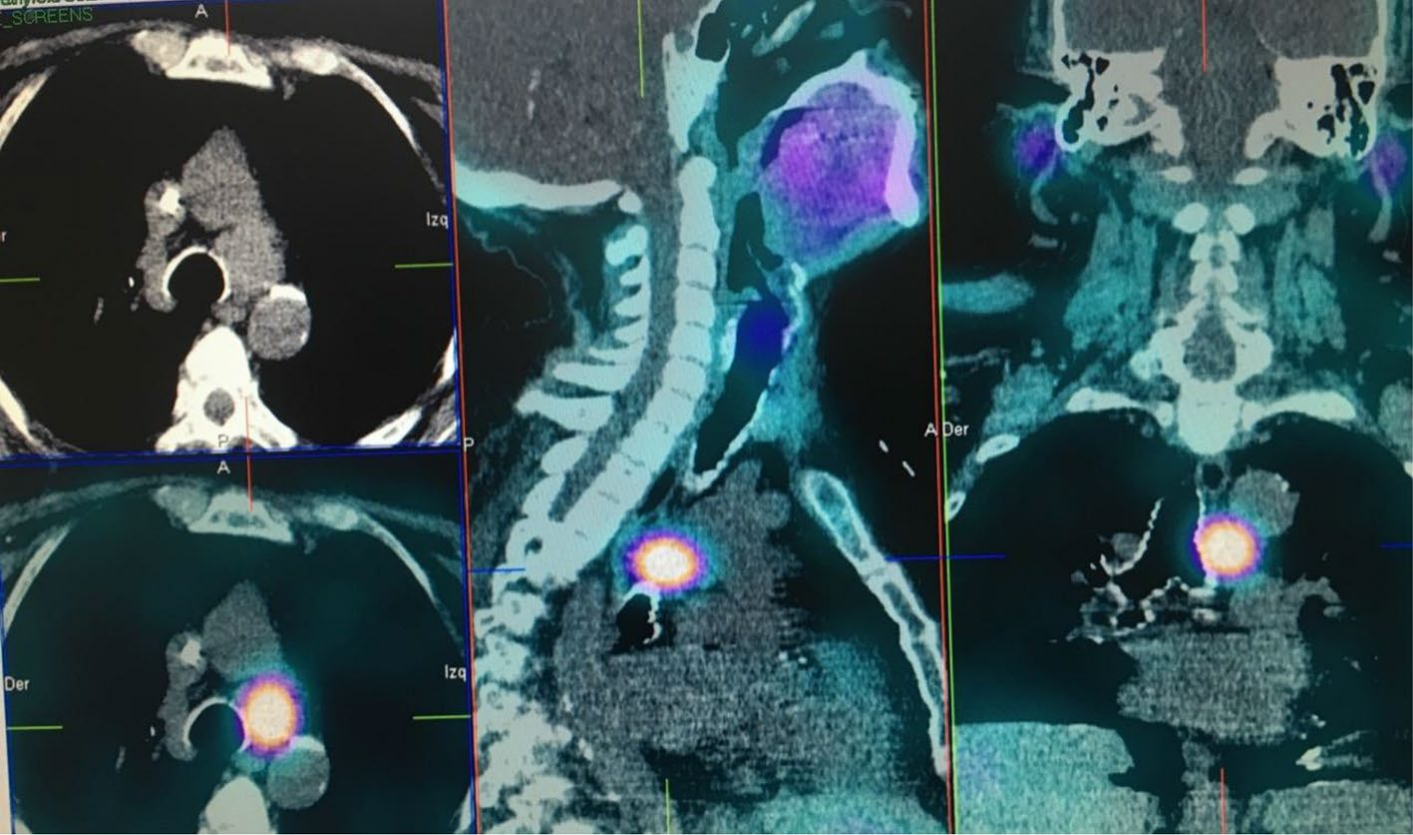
SPECT-CT scan with 99mTc Sestamibi in a patient with a MEPA in the aorto-pulmonary window
